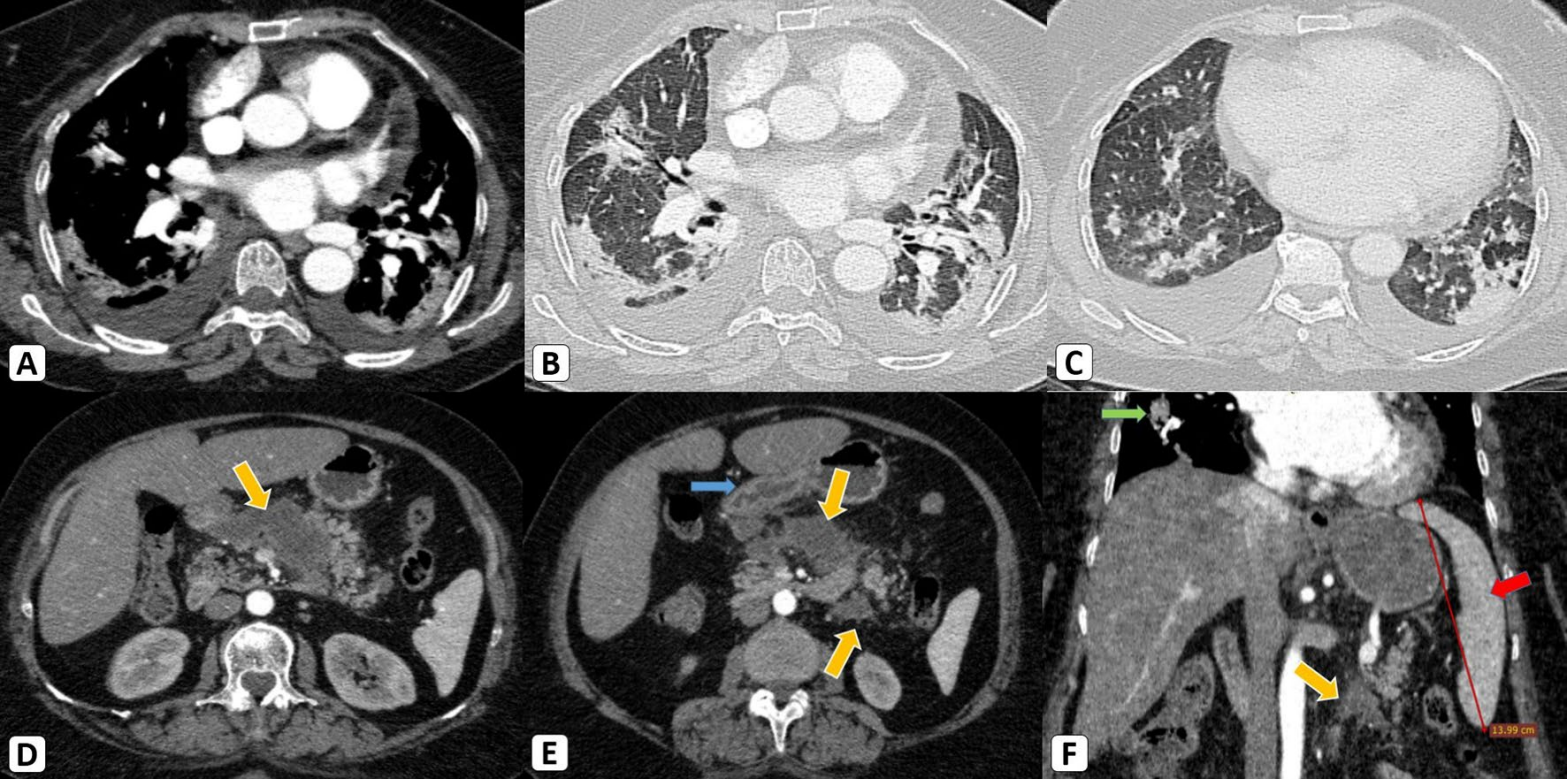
A–C Basal chest CT cuts (mediastinal and lung window) showed bilateral variable-sized bronchocentric and sub-pleural consolidative pneumonic patches (CO-RADS 5) with a mild bilateral pleural collection. D–E Axial abdominal CT cuts revealed a large peri-pancreatic and left anterior para-renal homogenous and hypo-attenuated fluid collections (8 Hu) with mild stranding of the nearby fat planes (yellow arrows). Nearby mural thickening and sub-mucosal edematous changes of the pyloro-duodenal junction, denoting secondary gastro-duodenitis (blue arrow). The pancreas retained homogenous parenchyma without necrotic areas. The main pancreatic duct (MPD) was not dilated. [F] Coronal abdominal CT cut revealed mildly enlarged spleen, reaching 14 cm in maximum bi-polar diameter, without focal lesions. The pancreatic collection (yellow arrow) and basal pneumonic changes (green arrow) are obvious *… COVID-19 infection complicated with acute pancreatitis and an acute peri-pancreatic fluid collection was the diagnosis according to the revised Atlanta Classification. The Balthazar modified CT-severity index (CTSI) was 6/10 (moderate)*
